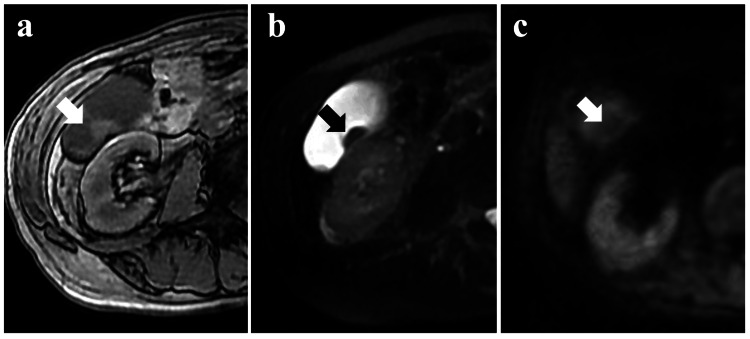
Abdominal magnetic resonance imaging The tumor shows iso-signal intensity on T1-weighted images (a) and low-signal intensity nodules on T2-weighted images (b). Low signal intensity is seen on diffusion-weighted images (c).
